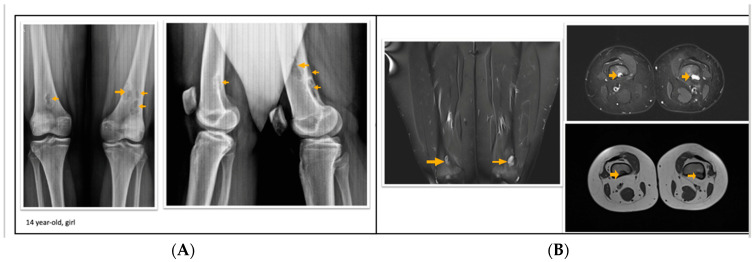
A 14-year-old girl with multiple fibrous cortical defects around the knee. (A) Direct X-ray of the knee in anteroposterior and lateral aspects show eccentrically located posteromedial and posterolateral lytic lesions with a sclerotic rim consistent with fibrous cortical defect. (B) Coronal and axial proton density and axial T1 weighted MR sections show lesions in posterior and posteromedial aspects of both femurs consistent with fibrous cortical defects. These lesions were classified as stage A according to Ritschl’s classification. Clinically, IPAQ (International Physical Activity Questionnaire): Moderately active, Screen Time: 3 h, 21-Numbered Circle VAS (21-VAS): 4.5, VAS (Visual analogue scale): 4, 21-Numbered Circle Activity Scale (21-NCAS): 6.
